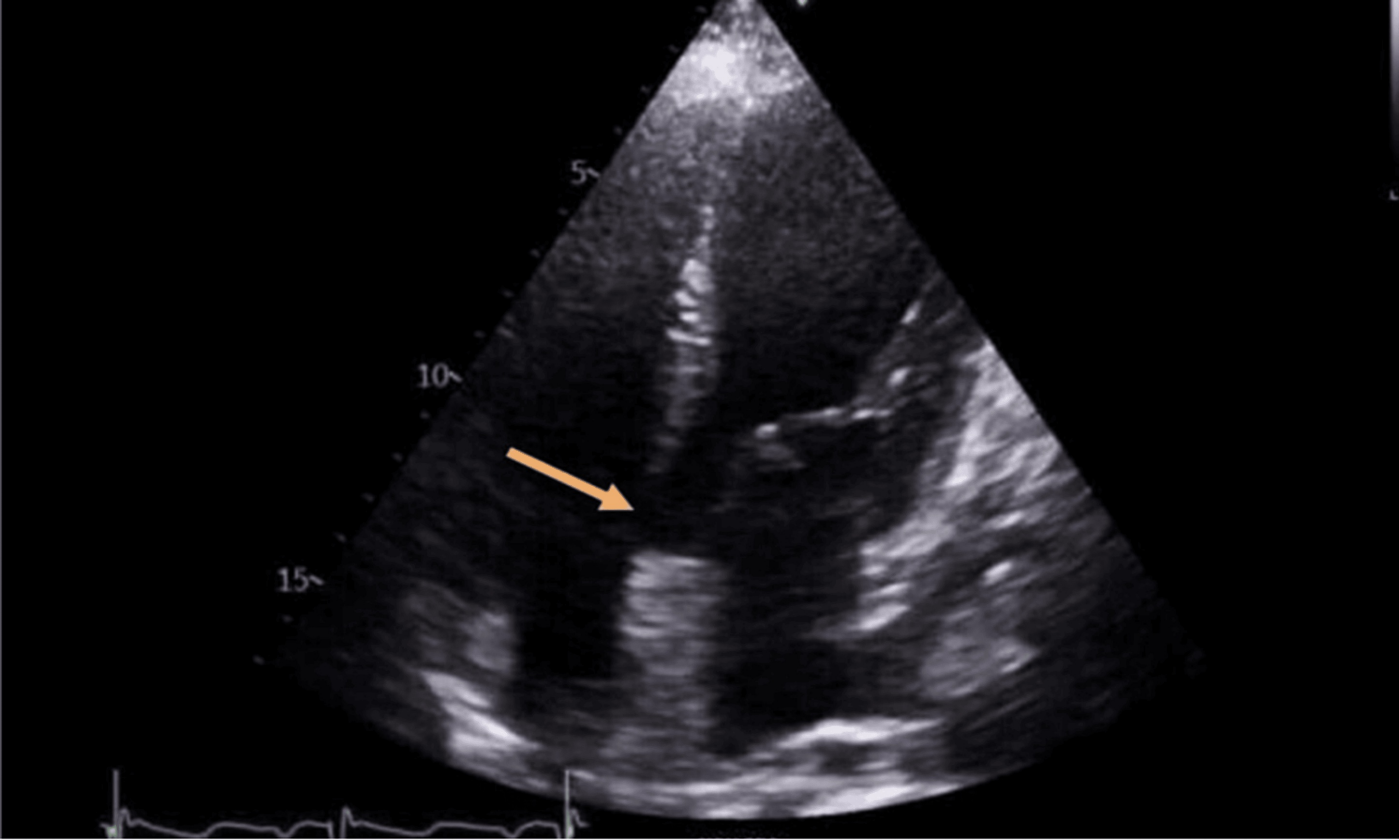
Yellow arrow shows a large membranous ventricular septal defect (VSD) in apical four-chamber echocardiographic view
